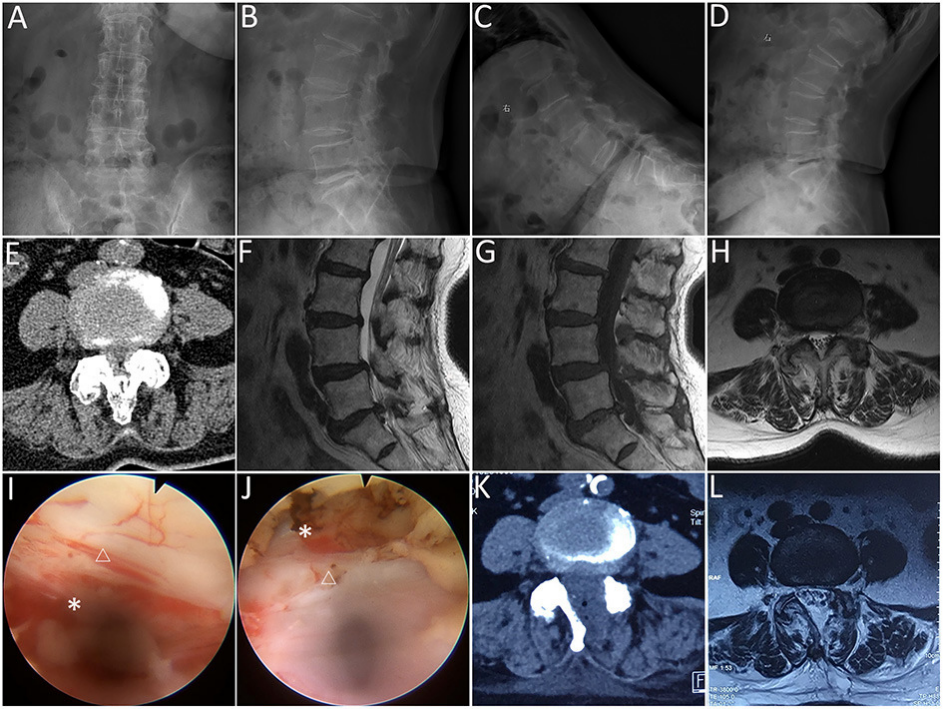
Lumbar endoscopic unilateral laminotomy bilateral decompression (LE-ULBD) performed on a 77-year-old female patient diagnosed with L4-L5 lumbar spinal stenosis with degenerative spondylolisthesis. (A,B) preoperative anteroposterior and lateral plain radiographs; (C,D) preoperative flexion and extension radiographs; (E) preoperative computed tomography (CT) scans; (F–H) preoperative magnetic resonance imaging (MRI) scans; (I,J) medial facetectomy was performed to decompress the lateral recess and ensure adequate decompression of the traversing nerve root; (K) postoperative CT scans; (L) postoperative MRI scans. Snowflake, nerve root, triangle, dural sac. * is used to tell the readers where is the nerve root.
